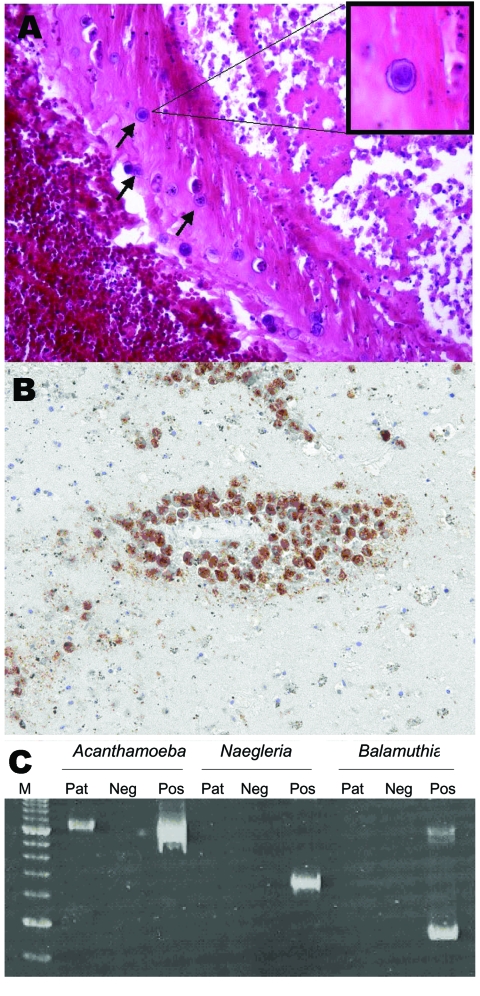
Magnetic resonance image of the patient’s brain after the first seizure showing a hemorrhagic lesion in the right frontal lobe.
